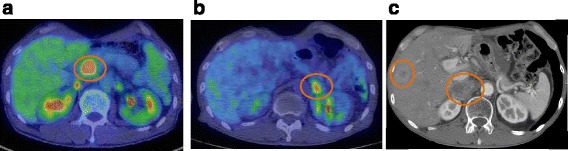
Recurrence findings. a Positron emission tomography-computed tomography scan findings of abdominal para-aortic lymph node recurrence at 3 years and 4 months after esophagectomy. b PET-CT scan of left adrenal gland recurrence at 4 years and 5 months after esophagectomy. c CT scan of liver and right adrenal recurrence at 5 years and 1 month after esophagectomy
